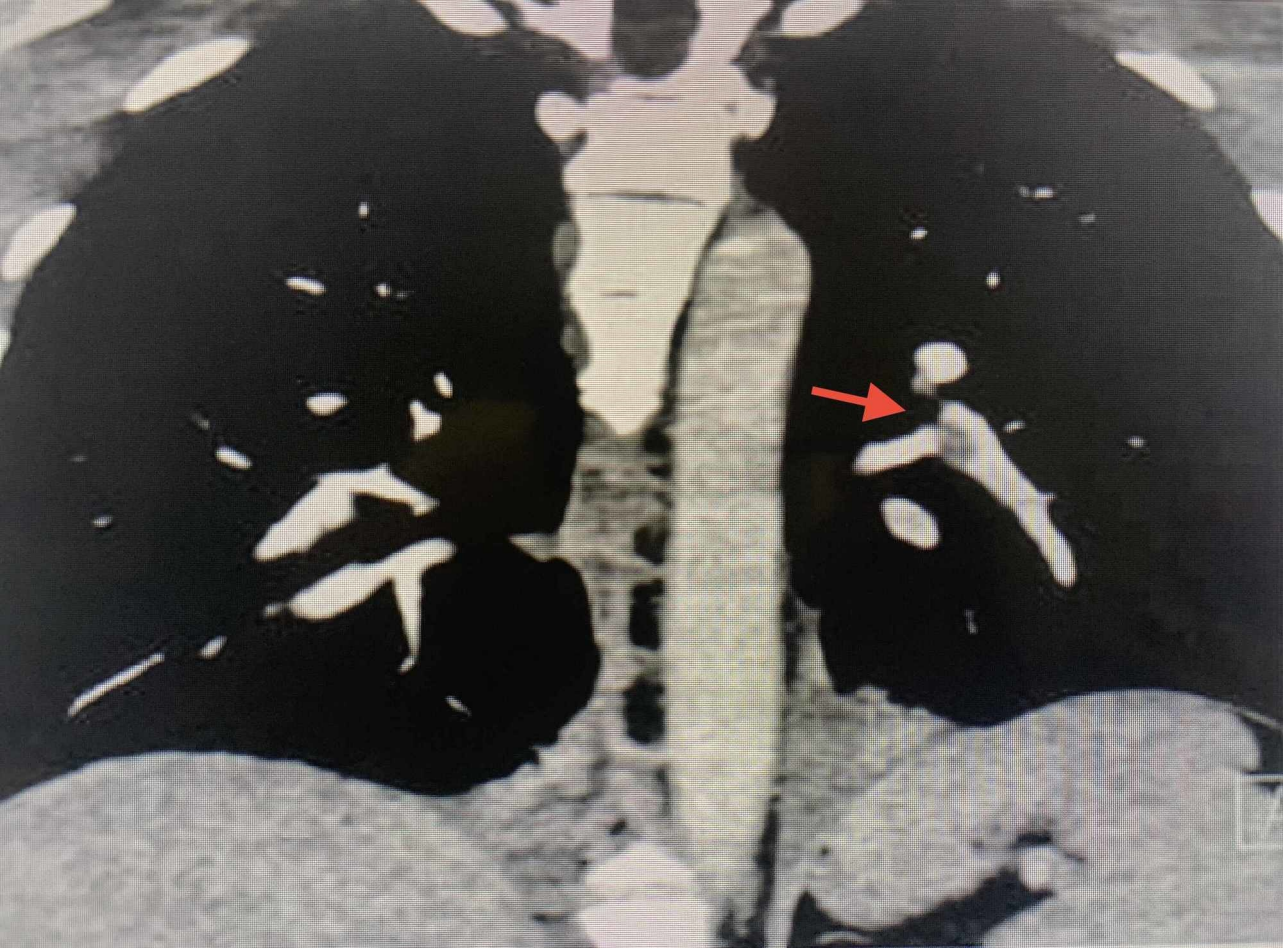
Coronal slice of the computed tomography pulmonary angiography showing segmental and subsegmental filling defects in the left pulmonary artery.
